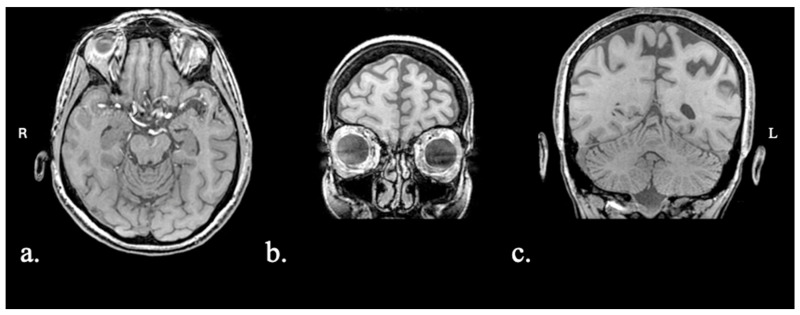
Patient FAY MRI scan: (a) axial view with asymmetrical (L > R) temporal atrophy; (b) coronal view with asymmetrical (L > R) frontal atrophy; (c) coronal view with asymmetrical (L > R) parietal atrophy (Scheltens 1, bilaterally).
